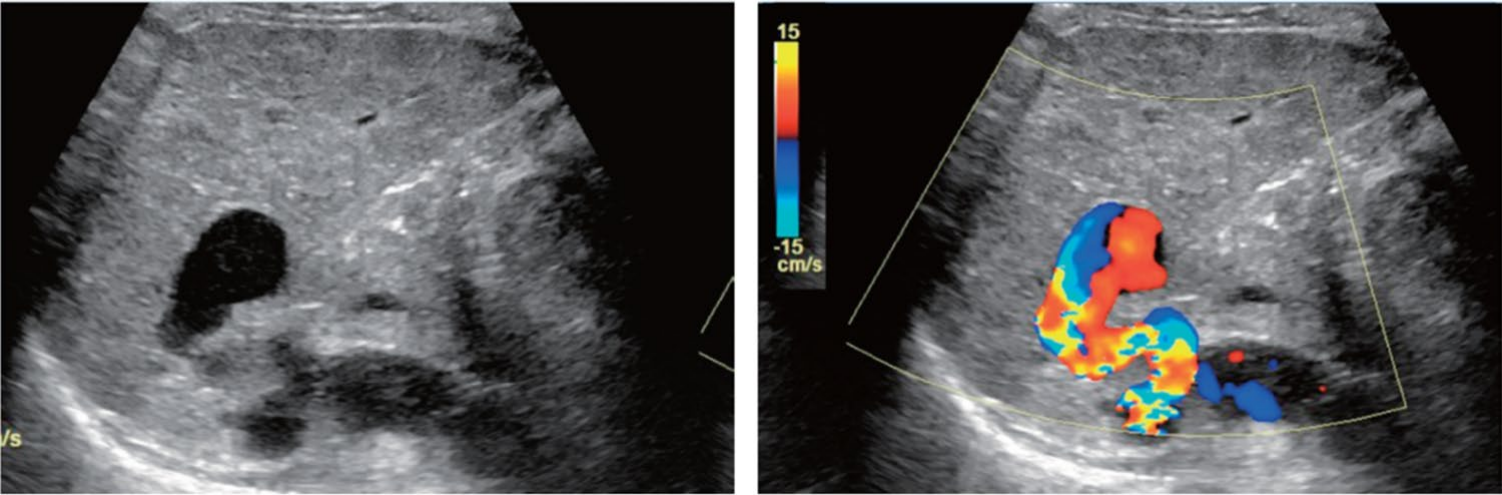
Other findings. Vascular abnormality (portal-venous shunt). Left: B-mode; right: color Doppler. Category 2, Assessment D2
